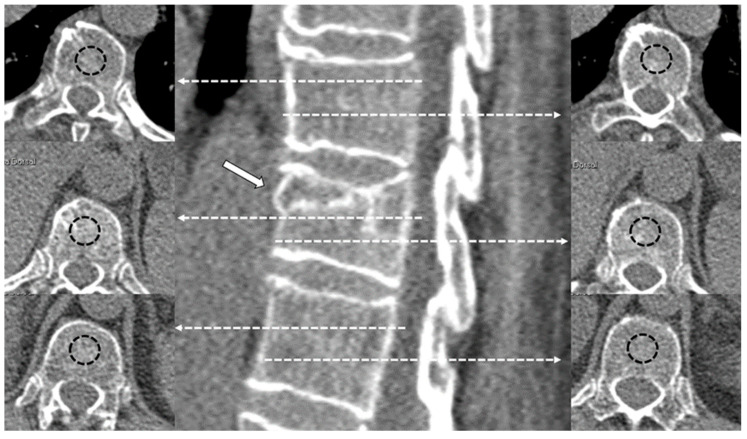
Examples of CT density measurements in a fractured vertebral body (arrow) and adjacent healthy vertebrae. The dashed lines with arrows indicate the level of different measurements in the axial plane. The dashed circles represent the region of interest (ROI) measured in the central part of the vertebral body.
